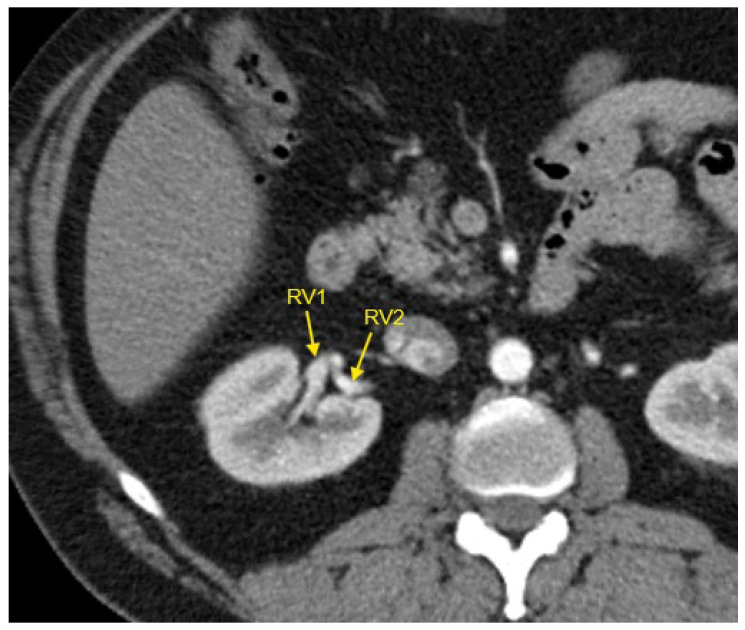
Contrast-enhanced CT imaging of a 44-year-old male hypertensive patient with difficult-to-control HTN revealed two renal veins in the right kidney, marked by the yellow arrows. CT—computed tomography; HTN—arterial hypertension; RV1—first renal vein; RV2—second renal vein.
